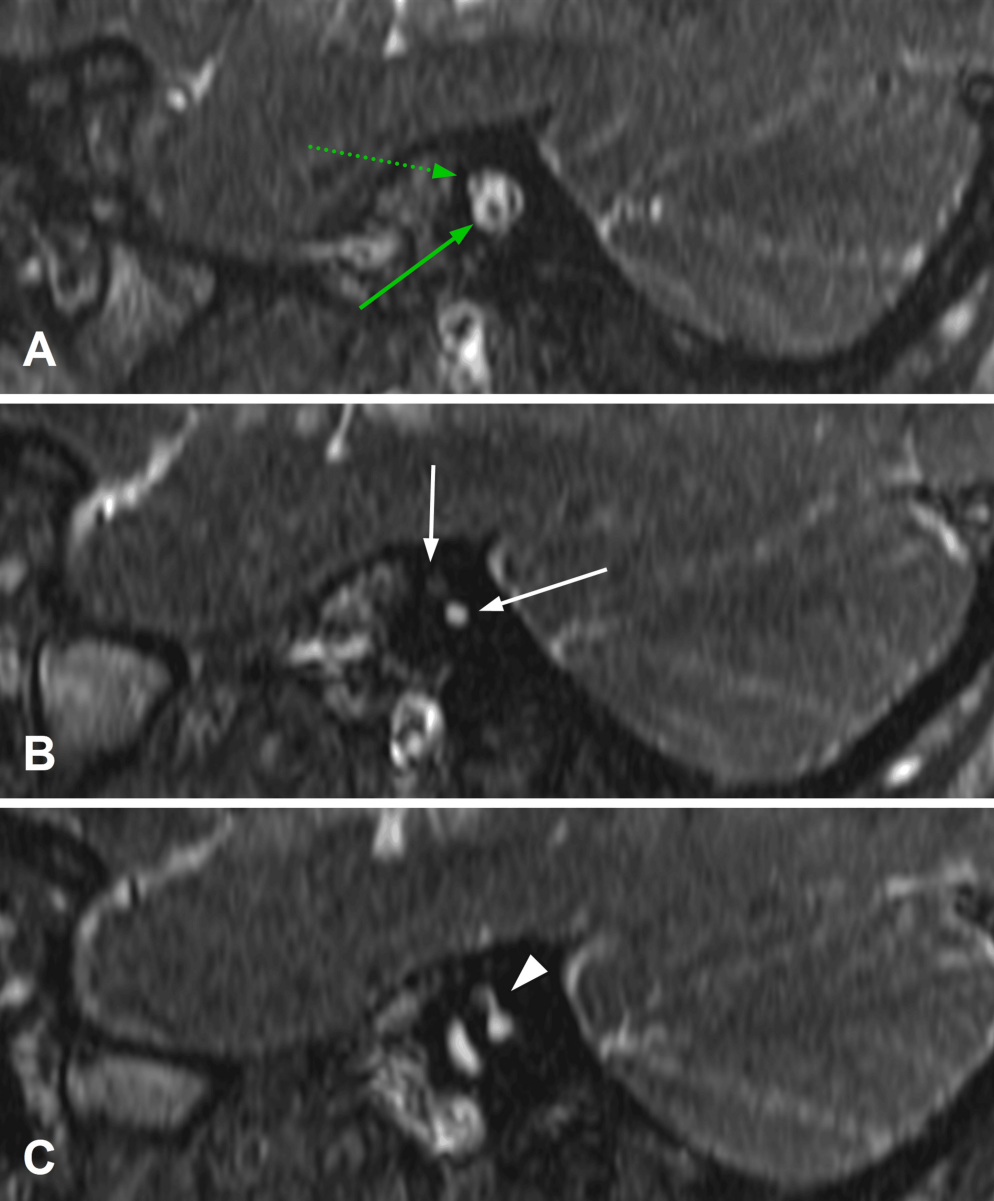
MRI oblique sagittal views: (A) apparent facial (dotted green arrow) and cochlear nerve (solid green arrow) traversing the left IAC; (B) duplication of the IAC on the right side without visualization of the cochlear nerve (white arrows); (C) distal merging of the previously separate DIAC forming the fundus MRI: magnetic resonance imaging; IAC: internal auditory canal; DIAC: duplicated internal auditory canal
